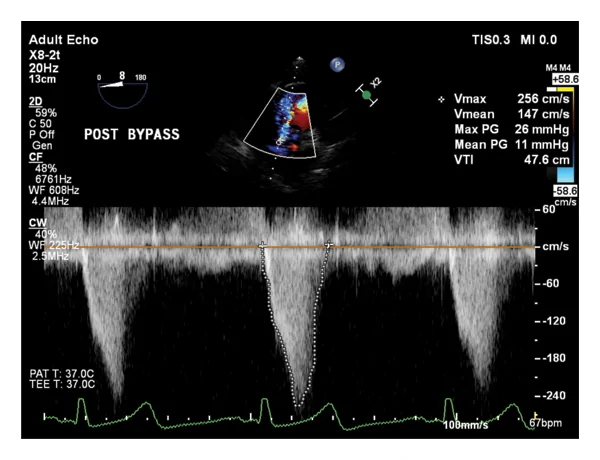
Deep transgastric TEE view with continuous‐wave Doppler across the LVOT and aortic valve, demonstrating a late‐systolic‐peaking, dagger‐shaped spectral envelope in a patient with SAM and dynamic LVOT obstruction.
